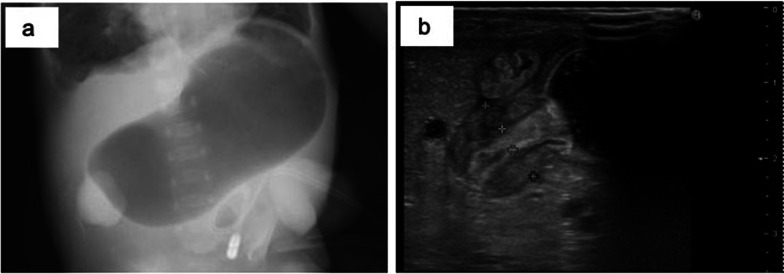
Abdominal radiography and ultrasonography findings at onset of hypertrophic pyloric stenosis. Distended stomach (a) and pyloric muscle thickened to 4-5 mm (b) are seen
